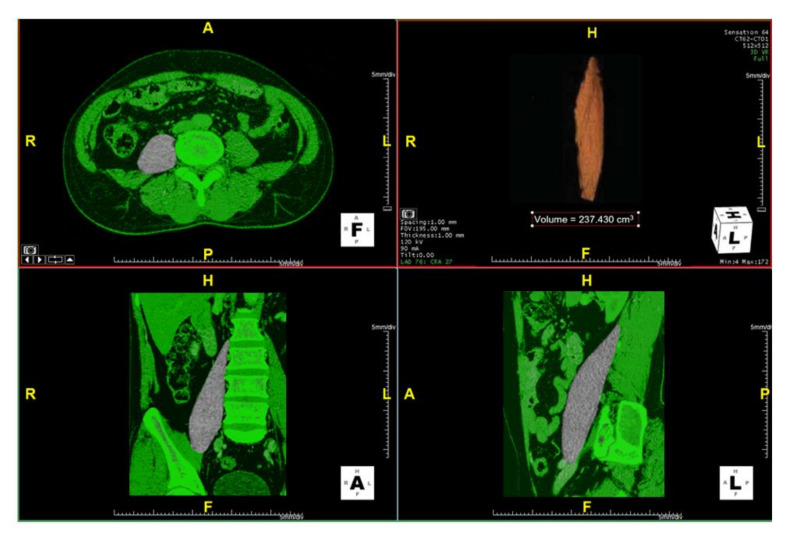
Images demonstrating the method of the psoas major muscle volume measurement by a CT-based software. Measurements are from the origin of the psoas major at the level of the lumbar vertebrae to its insertion in the lesser trochanter on CT datasets using a semi-automatic segmentation method. (A, anterior; P, posterior; R, right; L, left; H, head; F, foot).
